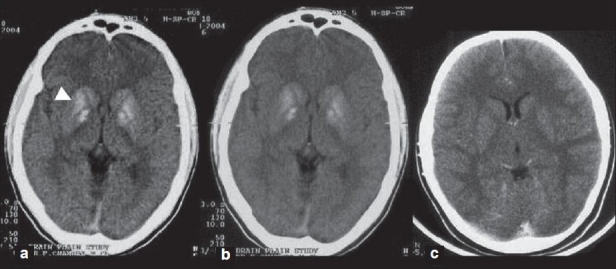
CT scans of the patients with pseudohypoparathyroidism showing striatopallidal calcifi cation in patient # 1 (a) and 2 (b) and the CT scan was normal in patient # 3 (c).
